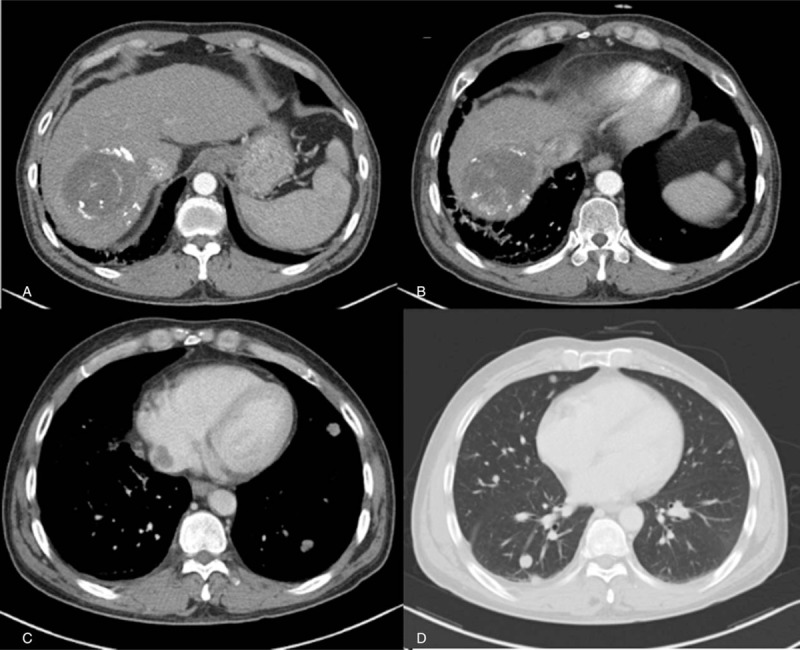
Follow-up abdominal computed tomography (3 months after diagnosis) (CT) revealed about 8.5 × 7.0-cm-sized decreased size of necrotic mass of hepatocellular carcinoma in right hepatic lobe (A), mildly decreased tumoral thrombosis in suprahepatic inferior vena cava (B) and decreased size of metastasis of right atrium (C). Chest CT showed multiple newly detected nodules in both lungs, suggesting pulmonary metastasis (D).
